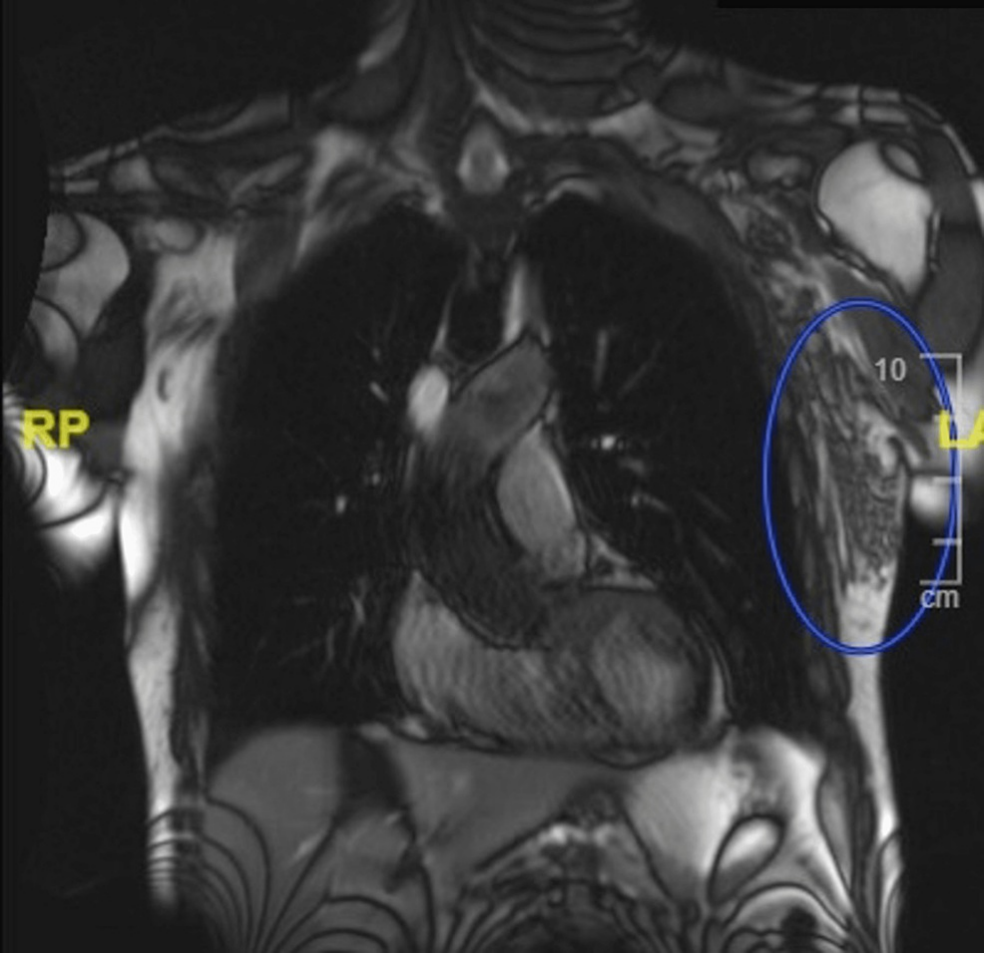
MRI of the chest Posttraumatic contusion in the left axilla with straining of the left latissimus dorsi, left subscapularis, left triceps, and biceps musculature. More confluent edema signal within the substance of the left latissimus dorsi and subscapularis muscles, suggestive of muscle tearing and intramuscular hematoma, as indicated by the blue circle. No acute fracture.
